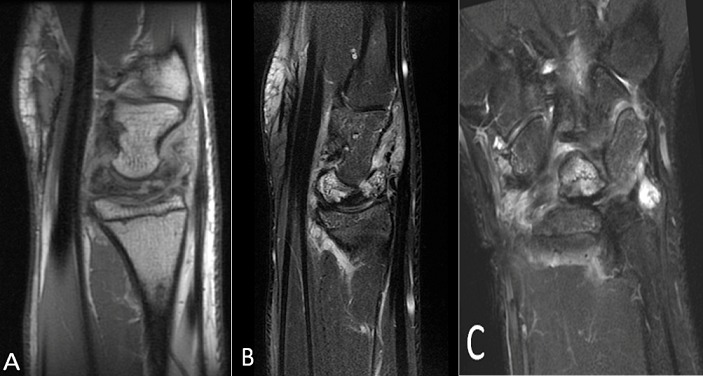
MRI of the left wrist: (A) sagital protons density showing collapse of the lunate with mixesd signal intensity; (B) sagital T2-weighted fat-suppressed MR image showing fragmented and irregular appearance of the lunate with hyper signal intensity; (C) coronal STIR showing fragmented and irregular appearance of the lunate with hyper signal intensity
